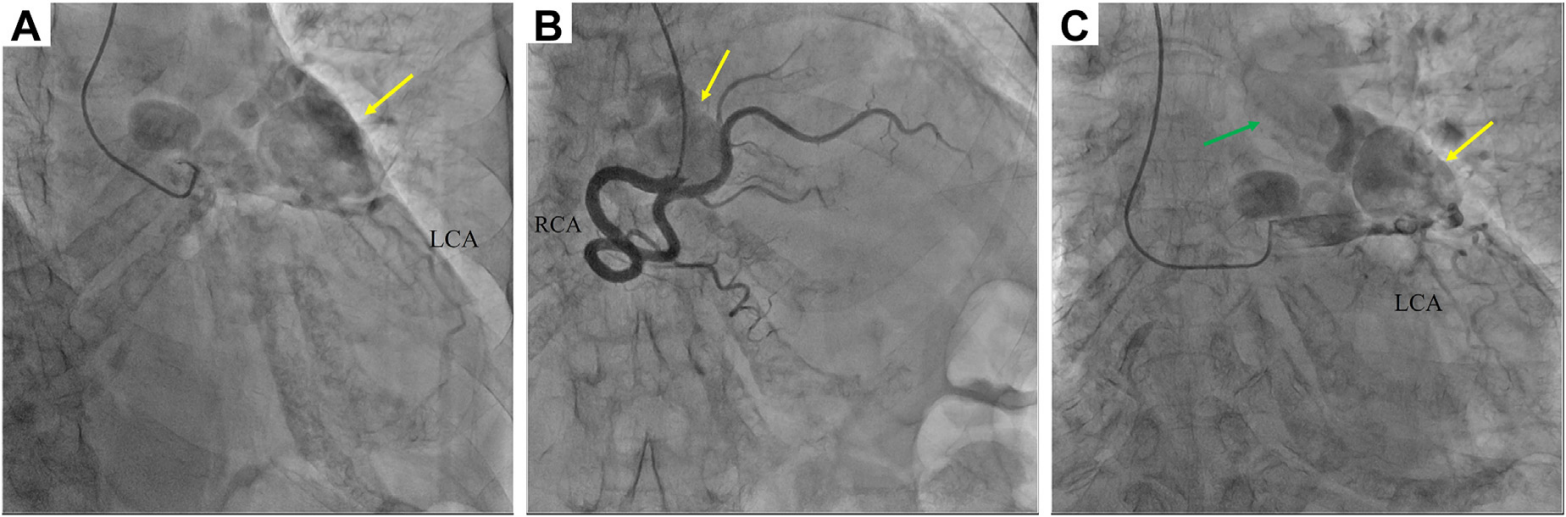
(A) and (C) show left coronary artery injected with X-ray contrast dye to show aneurysm. (B) shows right coronary angiography. Coronary angiography reveals a beaded giant coronary artery aneurysm (yellow arrow). Contrast enhancement of the pulmonary artery is observed (green arrow). (LCA, left coronary artery; RCA, right coronary artery.)
